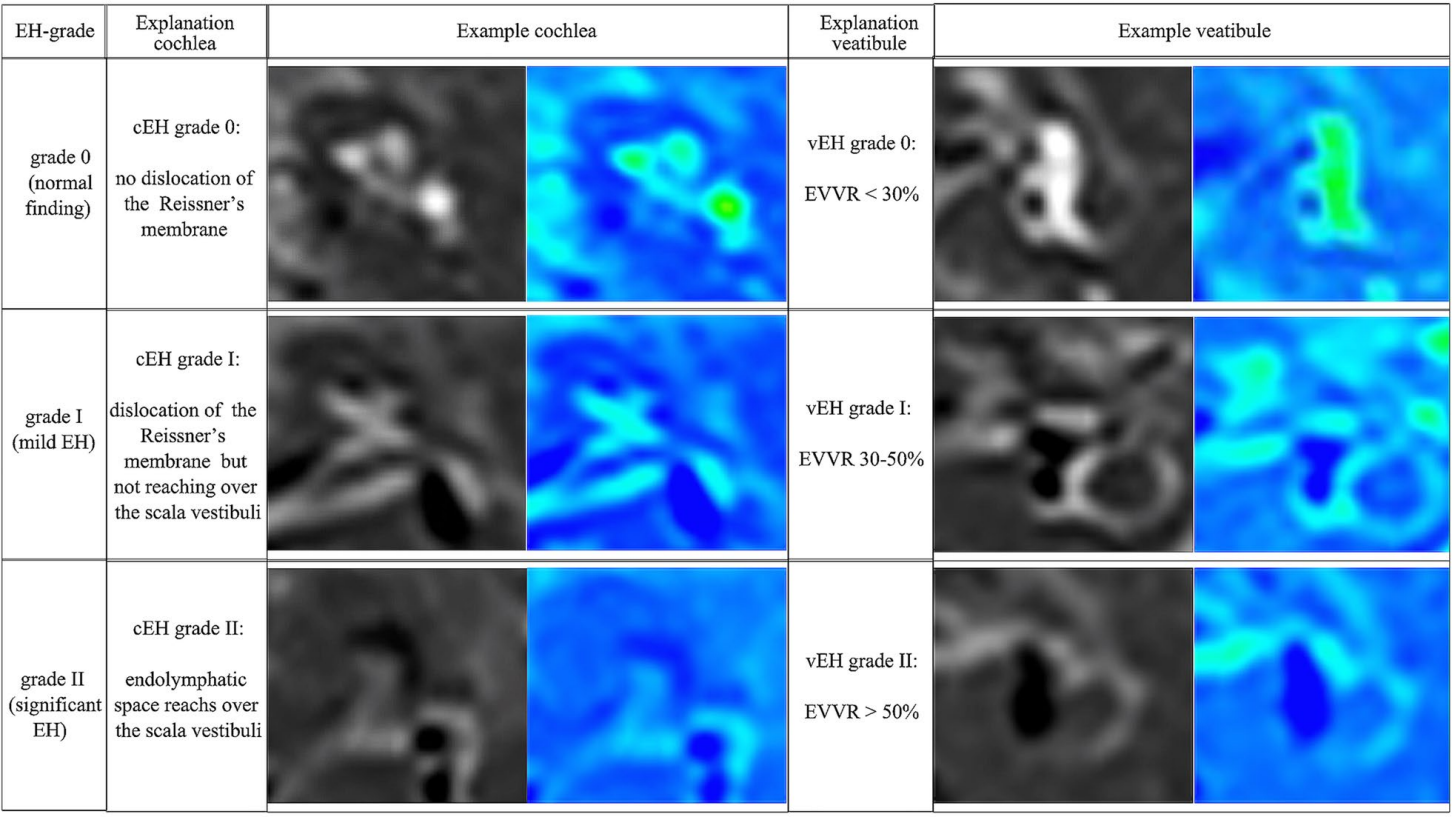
Criteria for grading of the cochlear and vestibular hydrops in three-dimensional fluid-attenuated inversion recovery MRI axial scans in the left column, and the corresponding MRI colored image in the right column. MRI, magnetic resonance imaging
